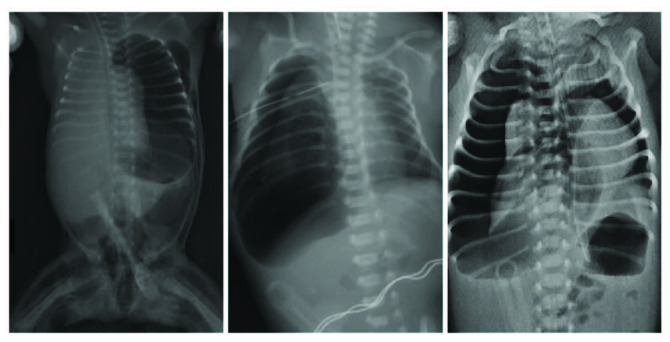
Radiographs of patients with type II pneumothorax.
